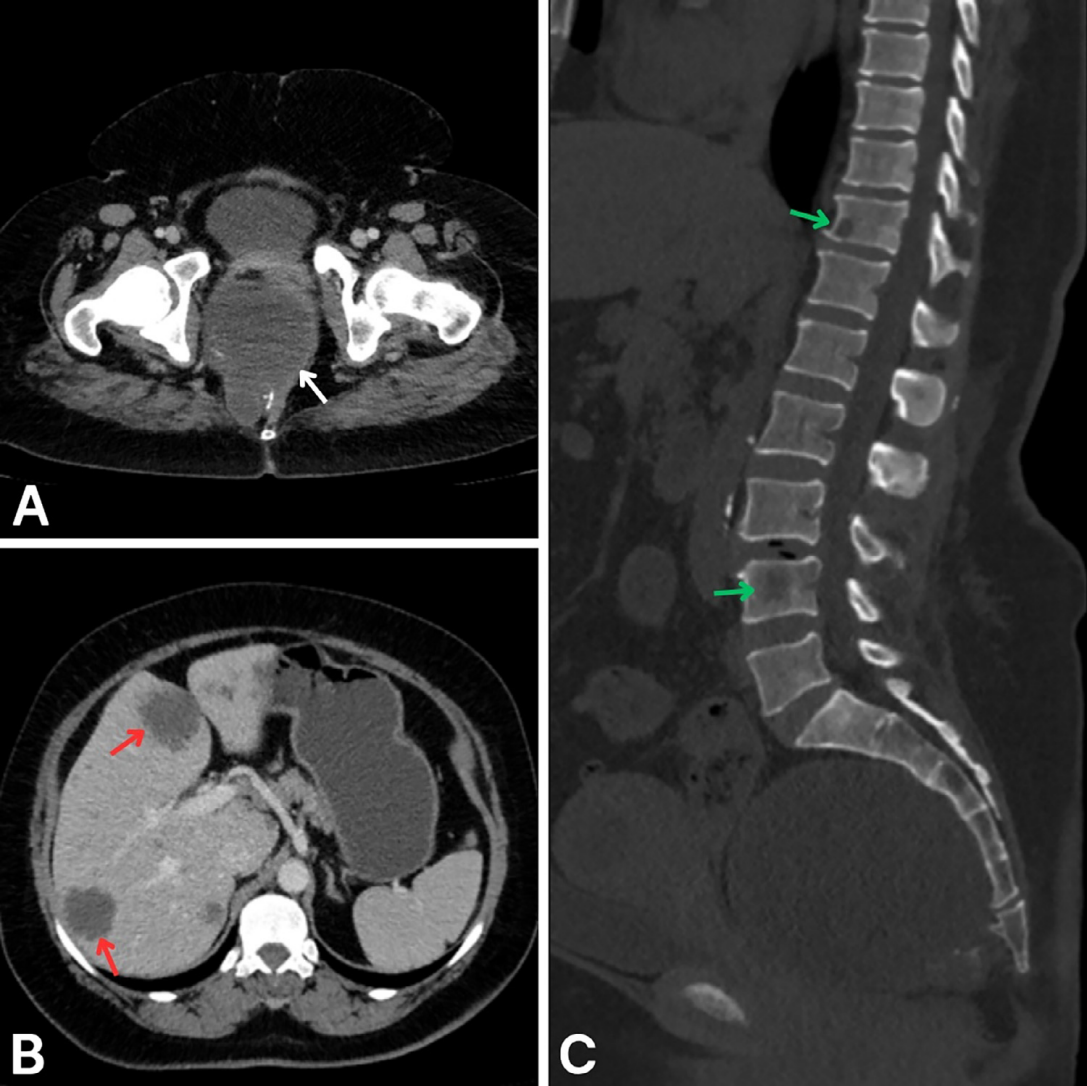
Follow-up abdominopelvic CT scan with and without intravenous contrast. (A) Axial CT at the pelvic level showing a presacral cystic lesion with peripheral nodular wall enhancement (white arrow). (B) Axial CT in the portal venous phase showing multiple hypodense hepatic lesions representing liver metastases (red arrows). (C) Sagittal CT in bone window showing lytic lesions at the D11 and L4 vertebral bodies, consistent with bone metastases (green arrows).
